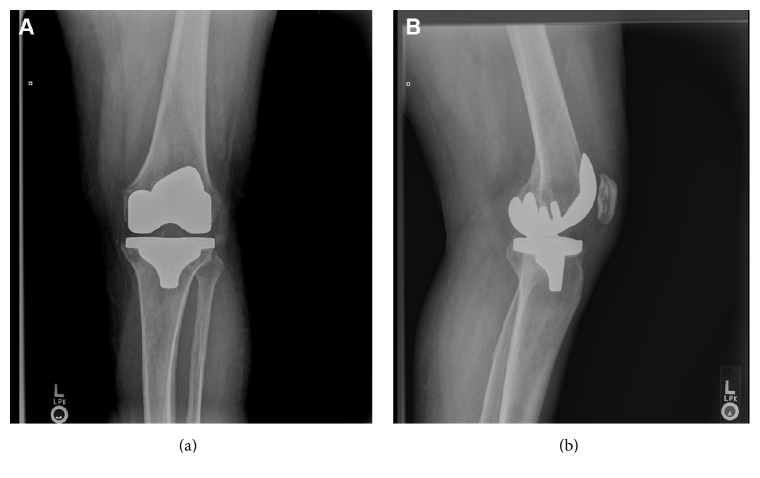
Anterior-posterior (a) and lateral (b) radiographs of left primary total knee arthroplasty from initial clinic presentation.
